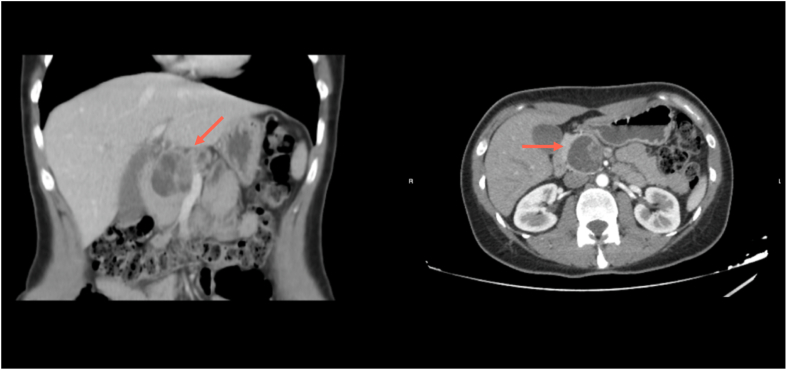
CT scan in which we observe the lesion of the pancreatic head.
